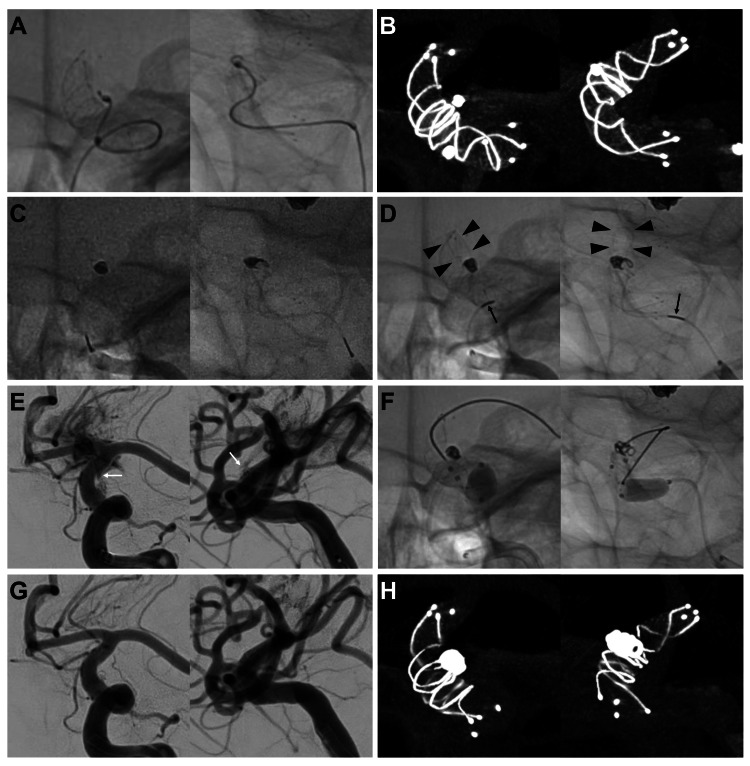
Imaging findings during endovascular surgery. The left side of each panel was the frontal working projection, and the right side of the lateral working projection. (A) Native imaging. A microcatheter was advanced into the aneurysm. One loop of a coil was inserted, and a stent was placed. (B) Cone beam CT with five-fold diluted contrast media shows an increased metal coverage of the aneurysm neck. (C) Native imaging. The coil was placed in the aneurysm, and the rear end of the coil alignment marker and the second microcatheter marker were matched. (D) Native imaging after coil detachment shows 9-mm advancement of the coil alignment marker (black arrows) compared to its position before detachment and advancement of the coil delivery wire (arrowheads) through the aneurysm wall. (E) Left internal carotid angiography after retrieving the perforated coil delivery wire showing extravasation of contrast media from the aneurysm (white arrow). (F) Native imaging. The balloon catheter was expanded at the proximal portion of the aneurysm. (G) Left internal carotid angiography after temporary balloon expansion shows no extravasation of contrast media from the aneurysm, and the aneurysm was occluded with a slight neck remnant. (H) Cone beam CT with five-fold diluted contrast media at the end of the surgery shows an increased metal coverage by shortening the proximal portion of the stent.
